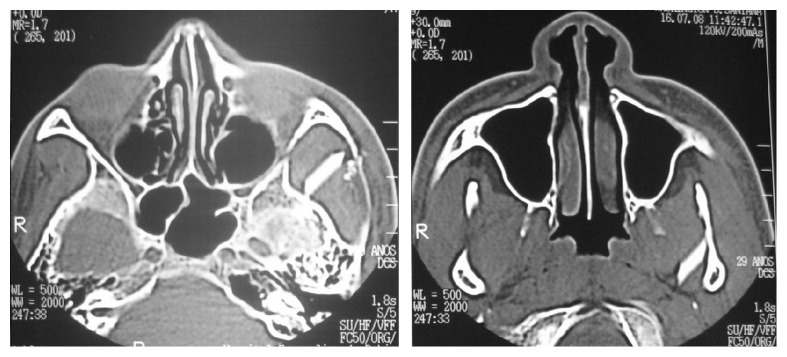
Computed tomography scan (hyperdense images medially to left zygomatic arch near to mandibular ramus).
